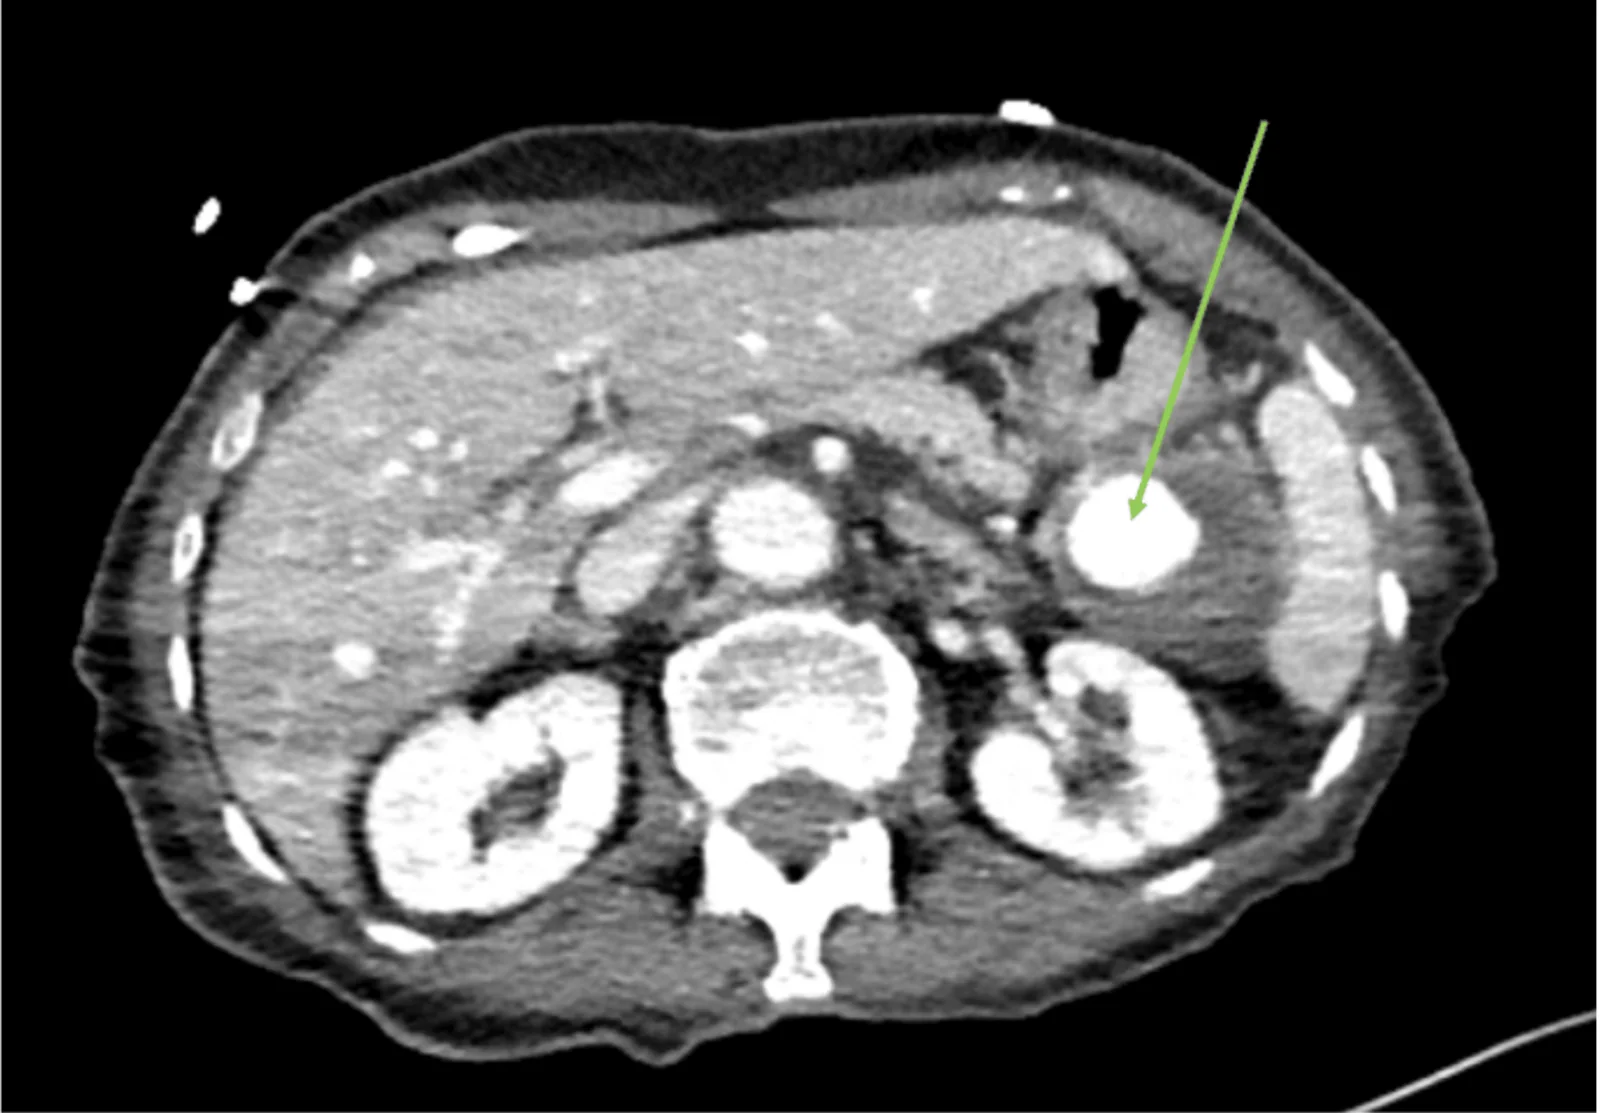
Axial arterial-phase contrast-enhanced CT scan of the abdomen The CT image is showing the SAP (arrow) with diffuse contrast opacification of the transverse colon, raising concern for a fistulous connection resulting in hemorrhage into the colonic lumen.
